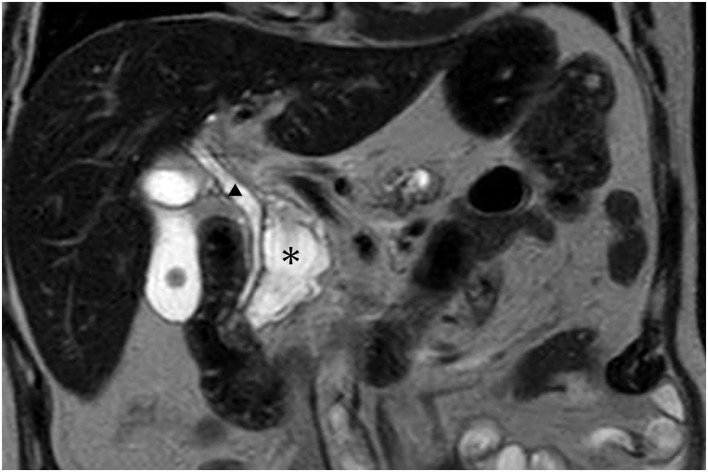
MRI image. MRI performed 1 month earlier showed no communication between the IPMN and the common bile duct (*; IPMN, ▲; common bile duct). IPMN, intraductal papillary mucinous neoplasm
